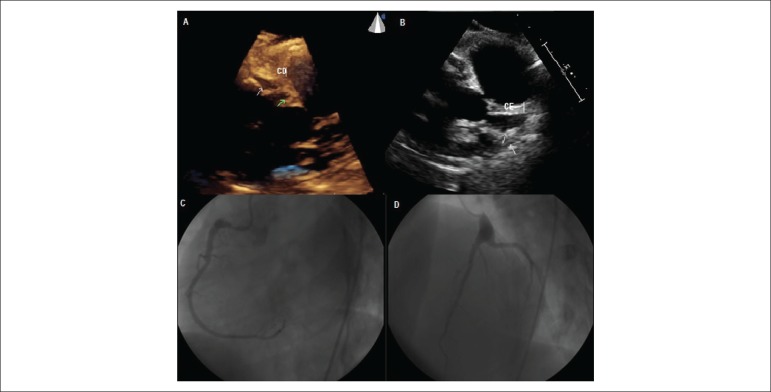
(A and B) Echocardiography: ejection fraction 68%; left atrium 35 mm;
